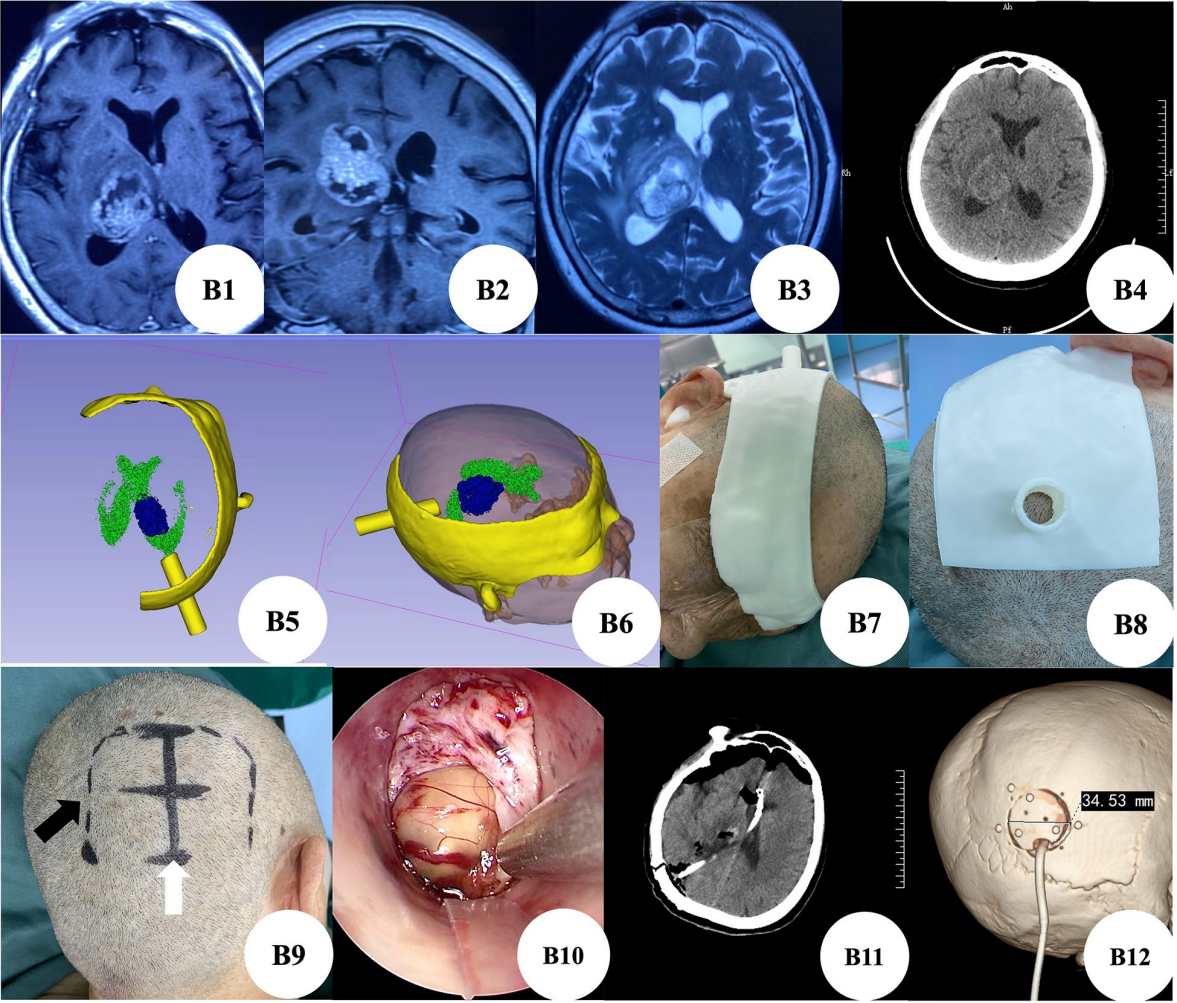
(B1–B3): Preoperative brain MRI showed space occupying lesions in the right thalamus. (B4): Preoperative brain CT showed space occupying lesions in the right thalamus. (B5–B6): 3D-Slicer reconstructed the relationship between the lesion and lateral ventricle and positioned the three-dimensional image of the guide plate. (B7–B8): 3D printing positioning guide. B9: The straight surgical incision (white arrow) is designed according to the printed positioning guide plate, and the black arrow is the conventional surgical incision. (B10): Lesions under transcranial neuroendoscopic. (B11): Postoperative brain CT showed that the lesion was resected completely. (B12): The three-dimensional reconstruction of the skull showed that the size of the bone window was about 3.5 cm.
